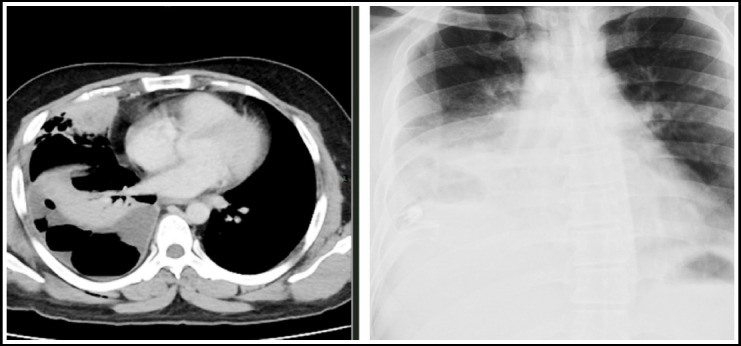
Chest X ray shows right pleural thickening with haze in the right lower lobe. Corresponding CT scan chest with IV contrast shows high density pleural effusion containing air. Diagnostic pleural tap showed frank pus.
